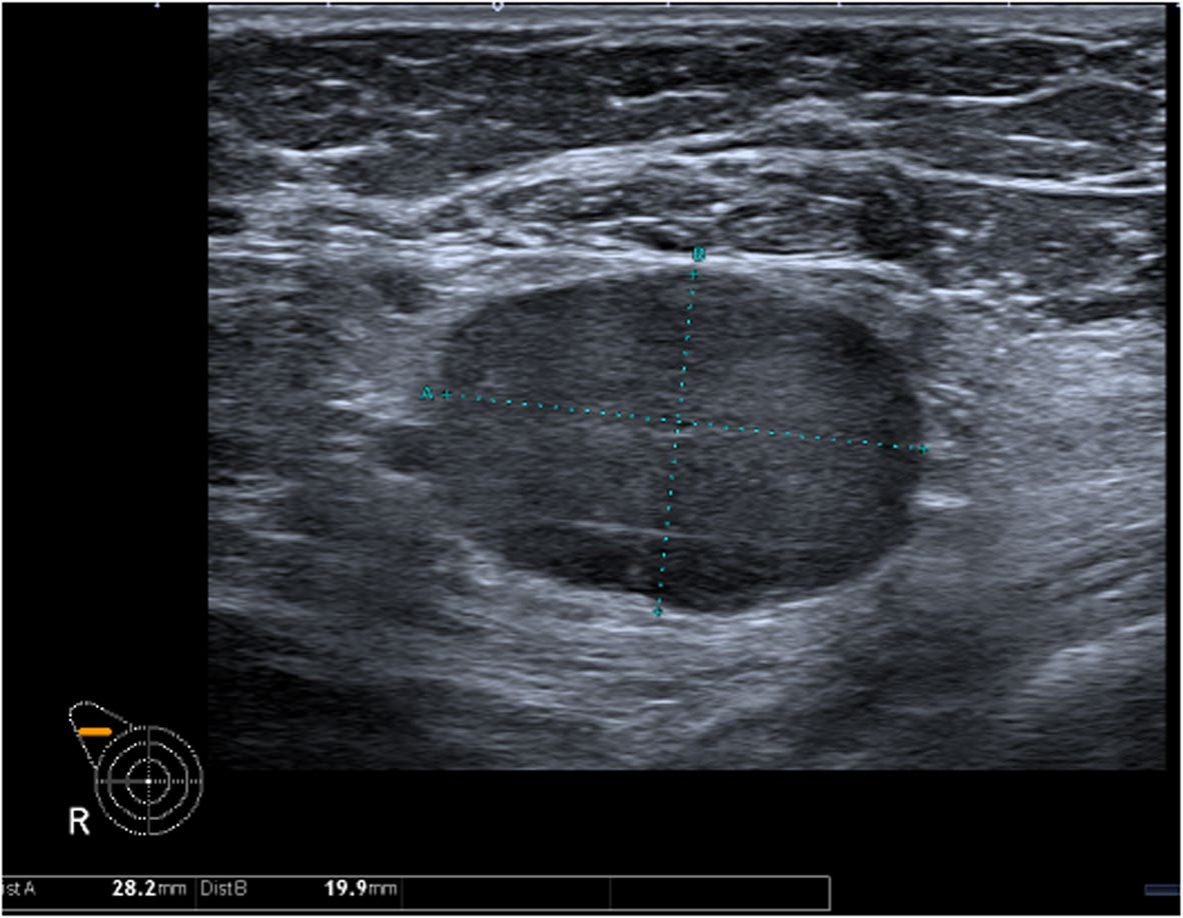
Pathological axillary lymph node on ultrasound before neoadjuvant systemic treatment measuring 28 × 20 mm
